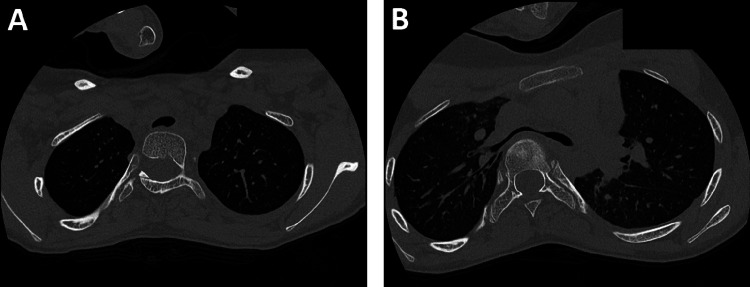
CT showing airway compression from thoracic lordosis. The CT of the chest taken as part of the preoperative assessment shows the compression of the distal trachea (A), carina and left and right main bronchi (B) caused by the vertebral bodies, as, in the presence of marked thoracic lordosis, the anteroposterior diameter of the chest had significantly decreased. CT: computed tomography
